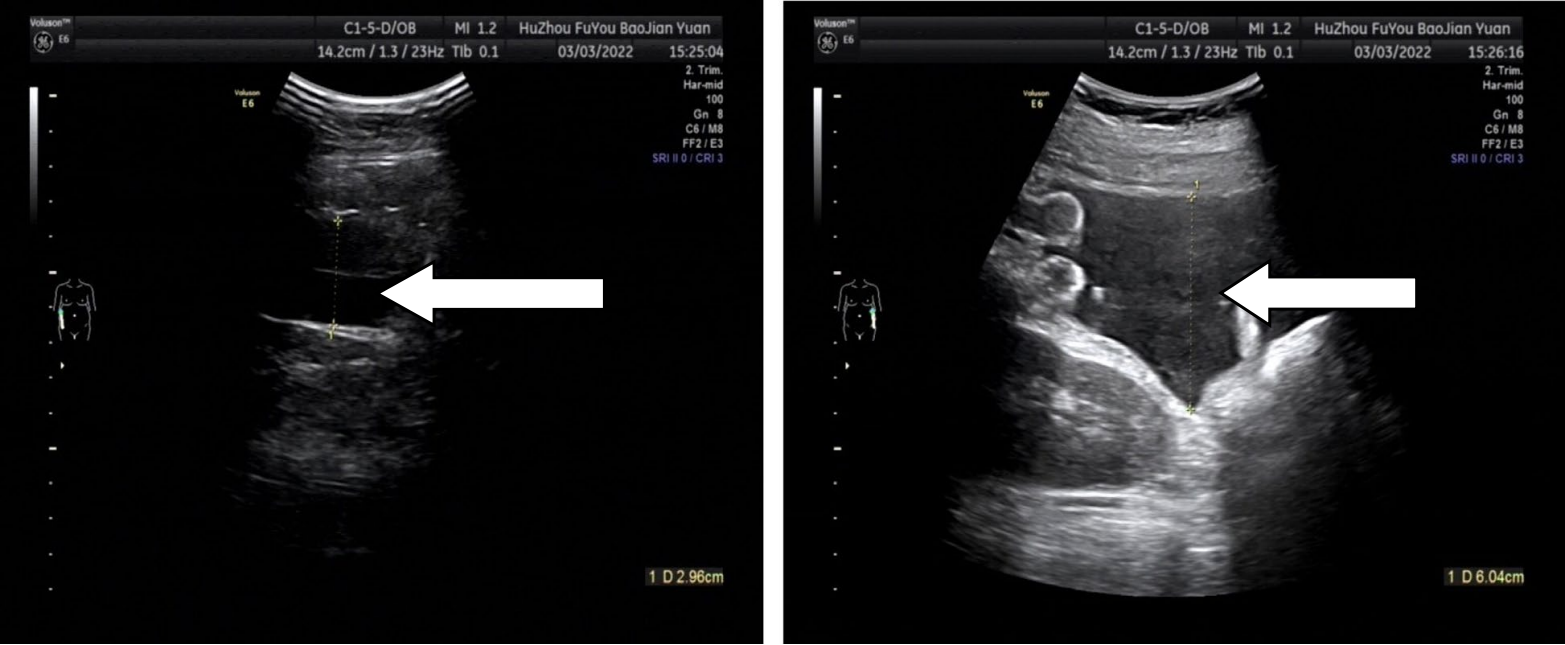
Ultrasonic findings indicating a large amount of peritoneal effusion
